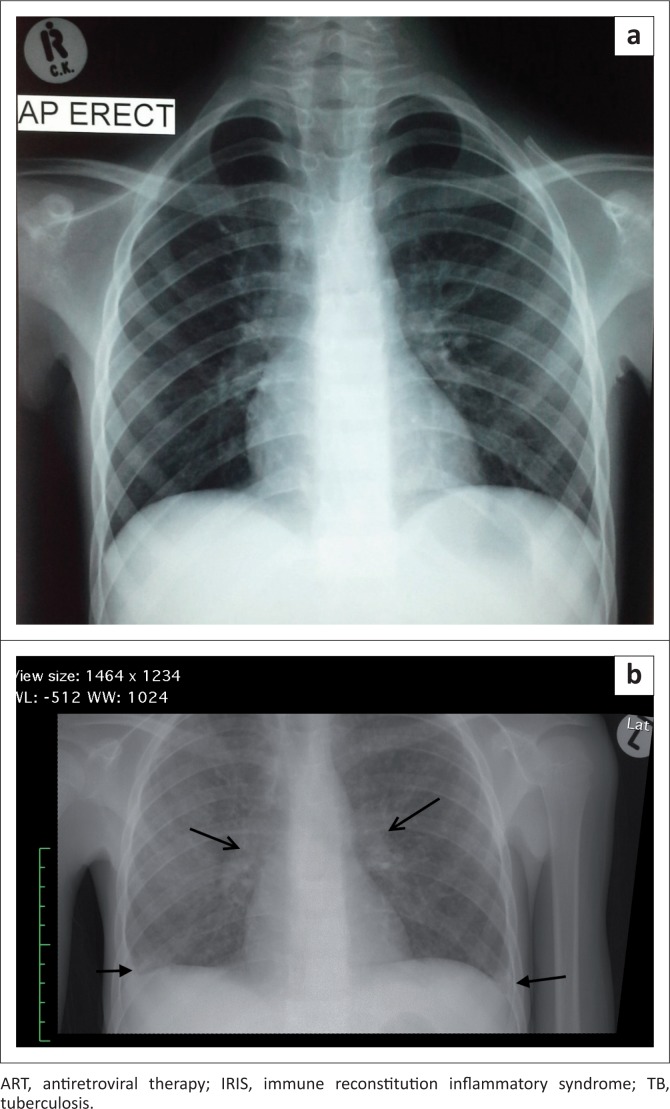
An 11-year-old HIV-infected boy, CD4 count 9 cells/mm^3^ (4%), was initiated on ART. He developed progressive symptoms consistent with disseminated TB and responded well to continuation of ART and standard four-drug TB treatment. Frontal chest radiograph taken at the time of ART initiation (a) demonstrates a normal chest radiograph. Frontal chest radiograph (b) taken 3 weeks after ART initiation demonstrates a diffuse reticular nodular infiltrate with bilateral hilar lymphadenopathy (open arrows) and small pleural effusions (closed arrows). A diagnosis of unmasking TB-IRIS was made.
